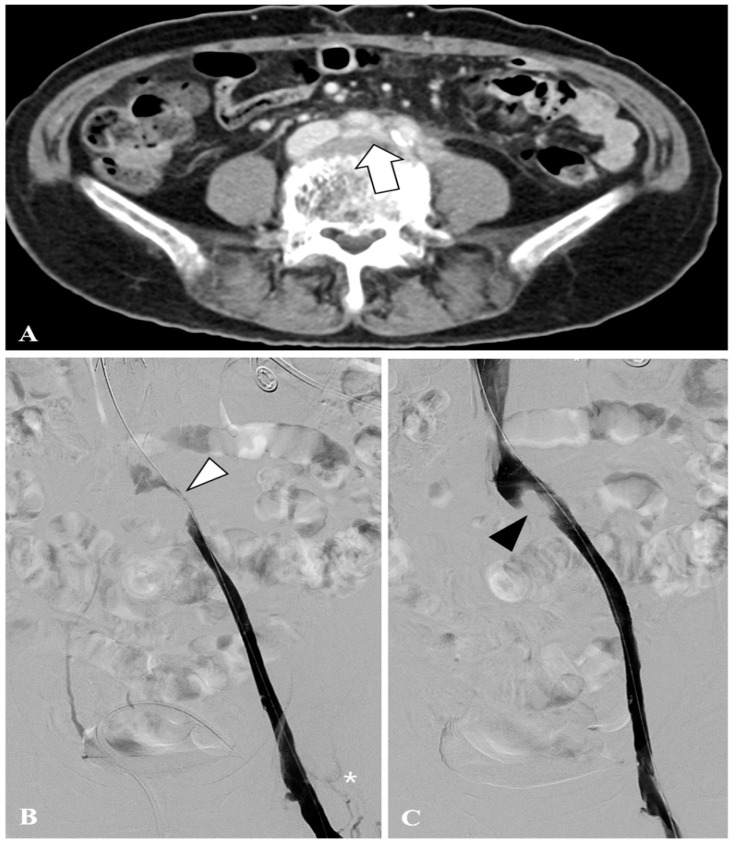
Endovascular treatment of May–Thurner syndrome-related iliofemoral deep vein thrombosis in a 66-year-old woman. (A) Contrast-enhanced computed tomography showing compression of the left common iliac vein (by the overlying right common iliac artery and L4 lumbar vertebral body (white arrow). (B) Conventional venography after thrombectomy demonstrates a severe stenosis (white arrowhead), along with collateral venous flow (asterisk). (C) Post-stenting venography shows partial relief of the stenosis; however, a residual luminal narrowing greater than 50% persists (black arrowhead). The collateral vein has resolved. This patient demonstrated stent reocclusion at the 1-month follow-up.
